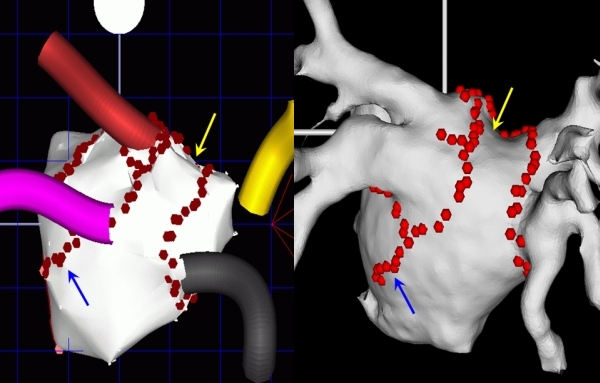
The electroanatomic map of the left atrium using CARTO (left) can be integrated with the CT/MRI images using CARTOMERGE Module (right). The arrows depict the corresponding points on the two maps.
